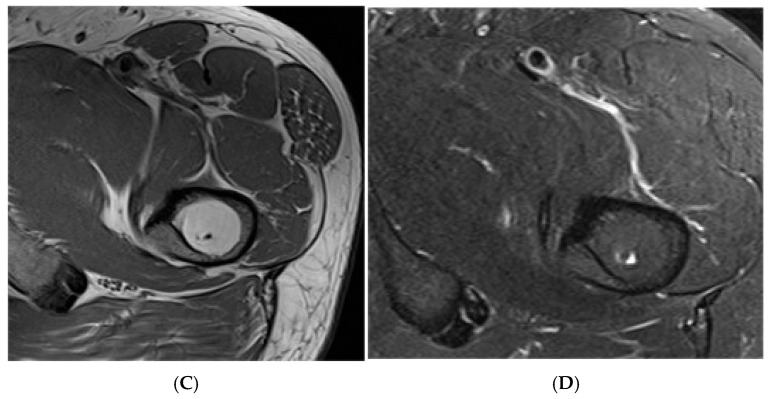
Intraosseous lipoma, left proximal femur. (A) AP radiograph proximal femur depicting well-defined, lucent bone lesion. (B) Coronal T1W MRI depicting fat-containing lesion. (C,D) Axial MRI T1W and STIR (respectively) demonstrating fat content without and with fat suppression.
